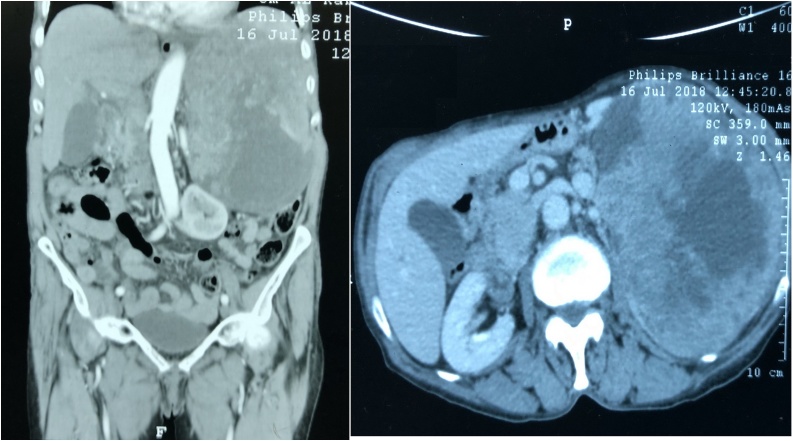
CT scan of the abdomen showing a huge mass that occupy the left upper abdominal cavity and displacing the left kidney, the mass has mixed cystic and solid components.
